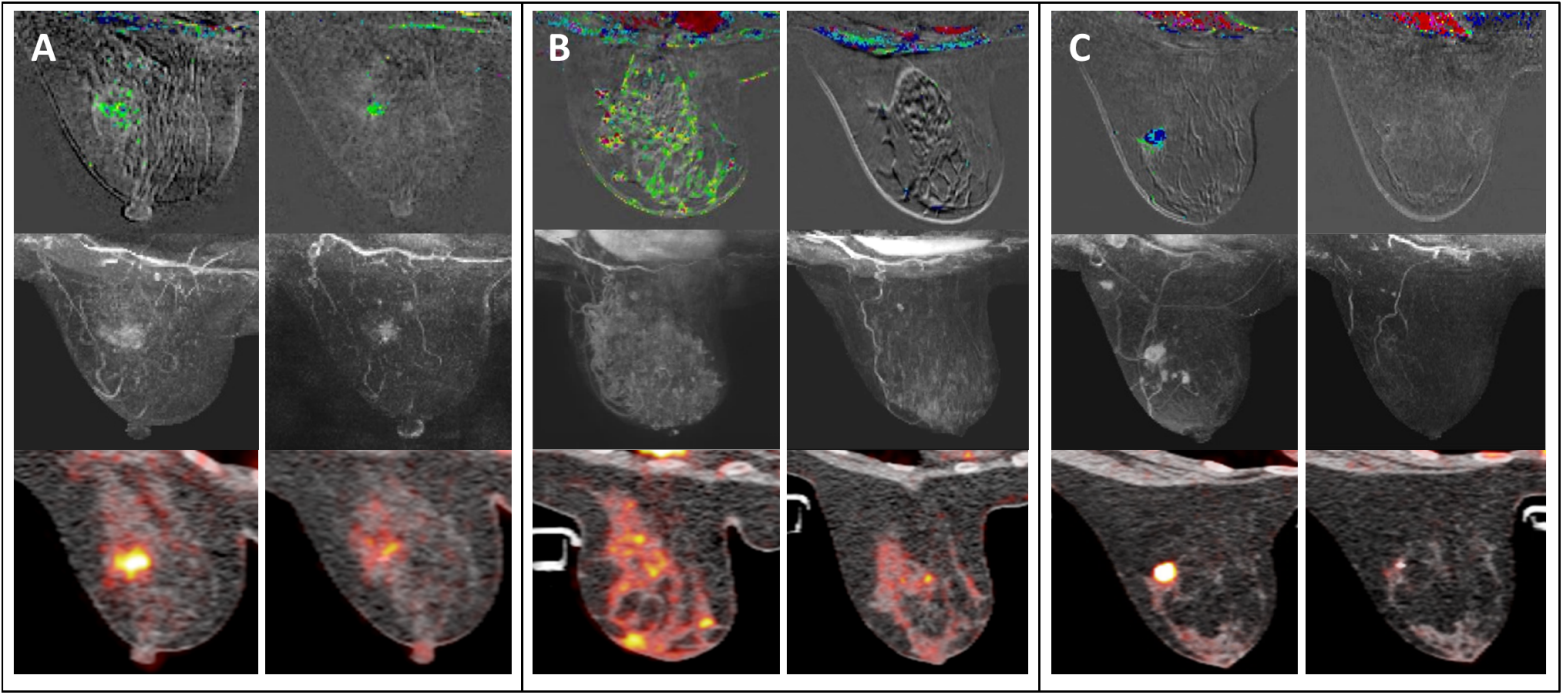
MRI and PET/CT imaging of different breast cancer subtypes. The top row shows MR subtraction images with color-coded visualization of contrast curves (persisting/green; plateau/blue; wash-out/red), the middle row shows maximum intensity projection of MR subtraction imaging, and the bottom row shows standardized uptake values on PET/CT imaging. For each example, imaging prior (left) and during (right) neoadjuvant chemotherapy is shown. (A) A 48-year-old women with an ER-positive invasive ductal carcinoma (IDC) showing a moderate response on MRI and PET/CT imaging, with a non-pathologic complete response (non-pCRmic) on final pathology. (B) A 52-year-old woman with a HER2-positive IDC showing a good response on MRI but a moderate response on PET/CT imaging, with a pCRmic on final pathology. (C) A 28-year-old woman with a triple-negative IDC showing a good response on MRI and PET/CT, with a pCRmic at final pathology.
